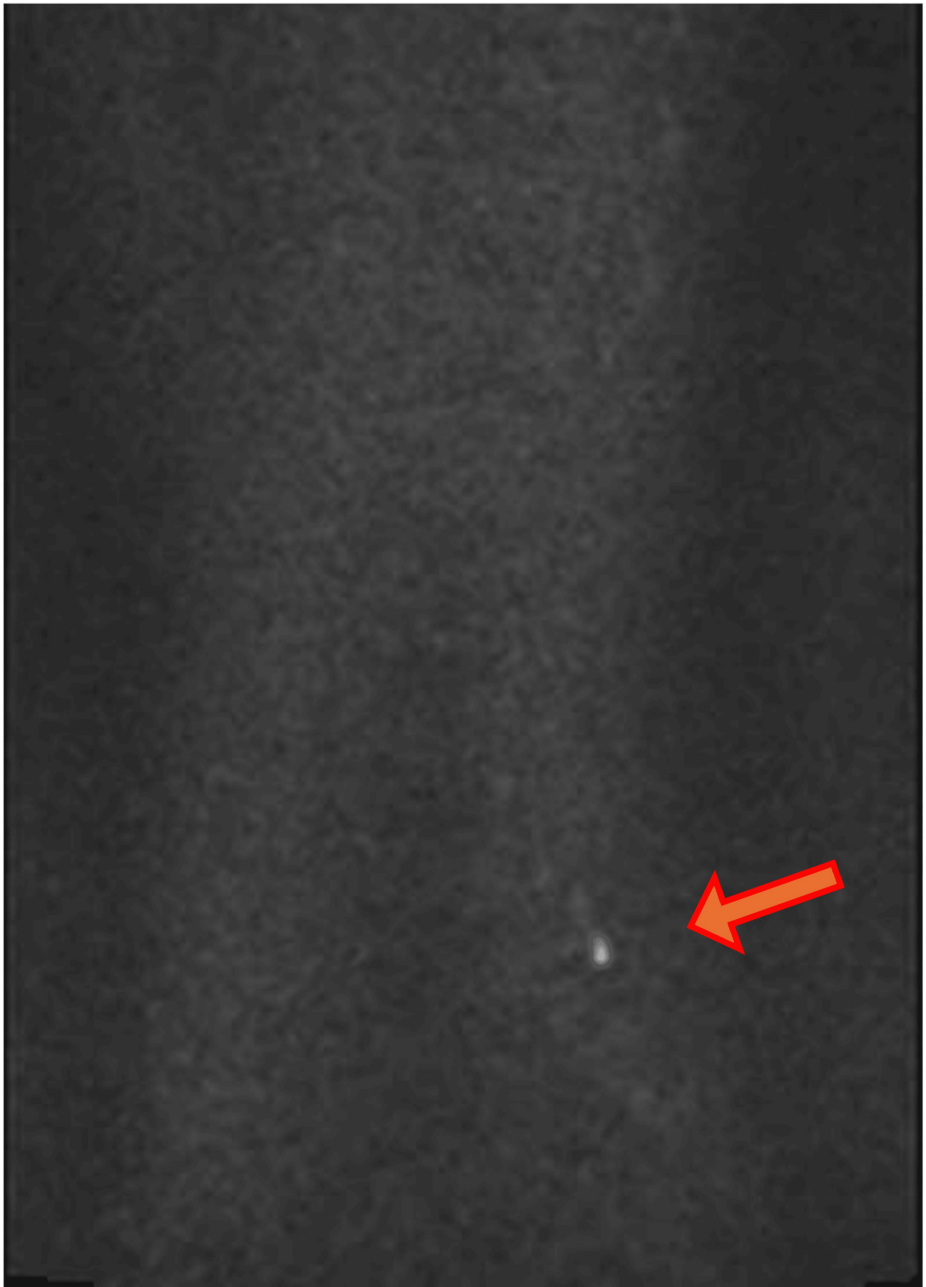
The DWI MRI at her first presentation This is a sagittal section taken with a DWI MRI at the first hospital admission. Although a small area of high signal (red arrow) was observed around L5/S1, clinical relevance was not appreciated. DWI: diffusion-weighted imaging
